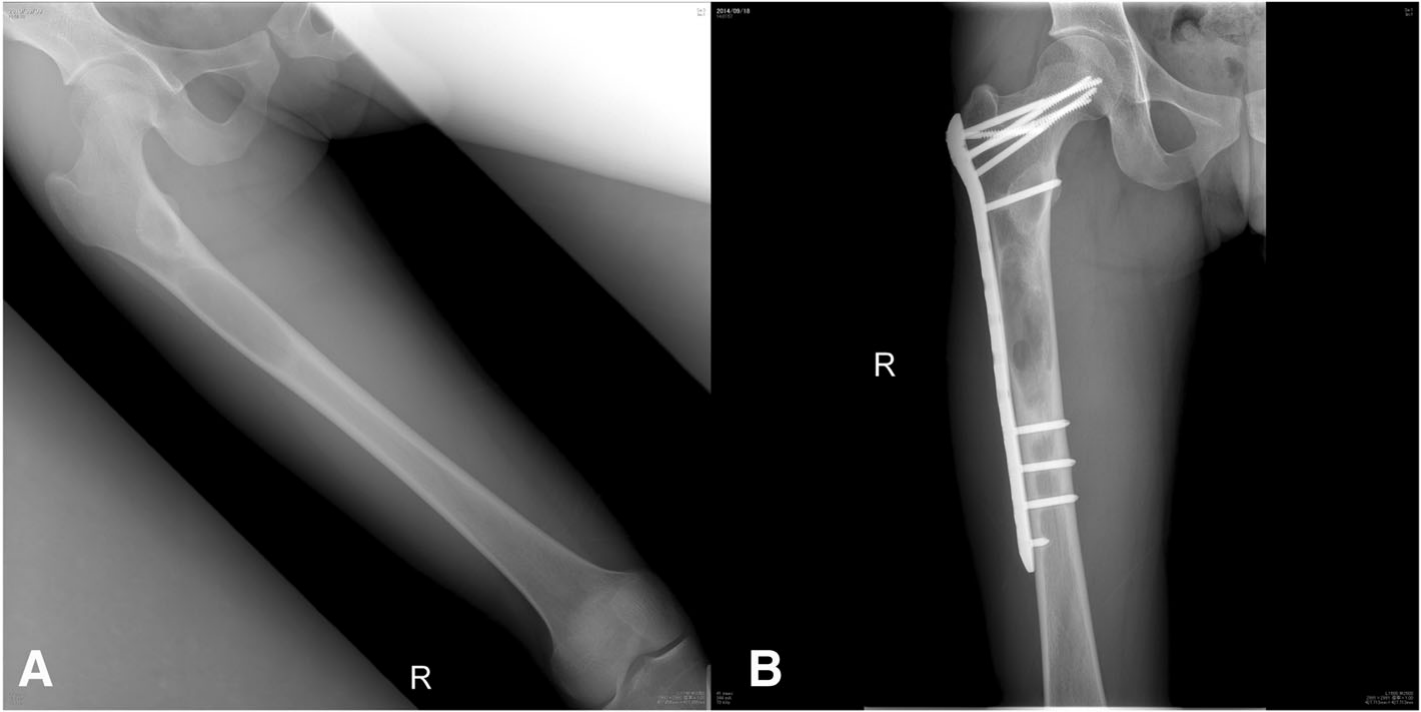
Patient 11: a AP radiograph showing femoral diaphyseal lesion. b Postoperative AP radiograph at 205 weeks after prophylactic bridge plating, showing partial resolution of the lesion
